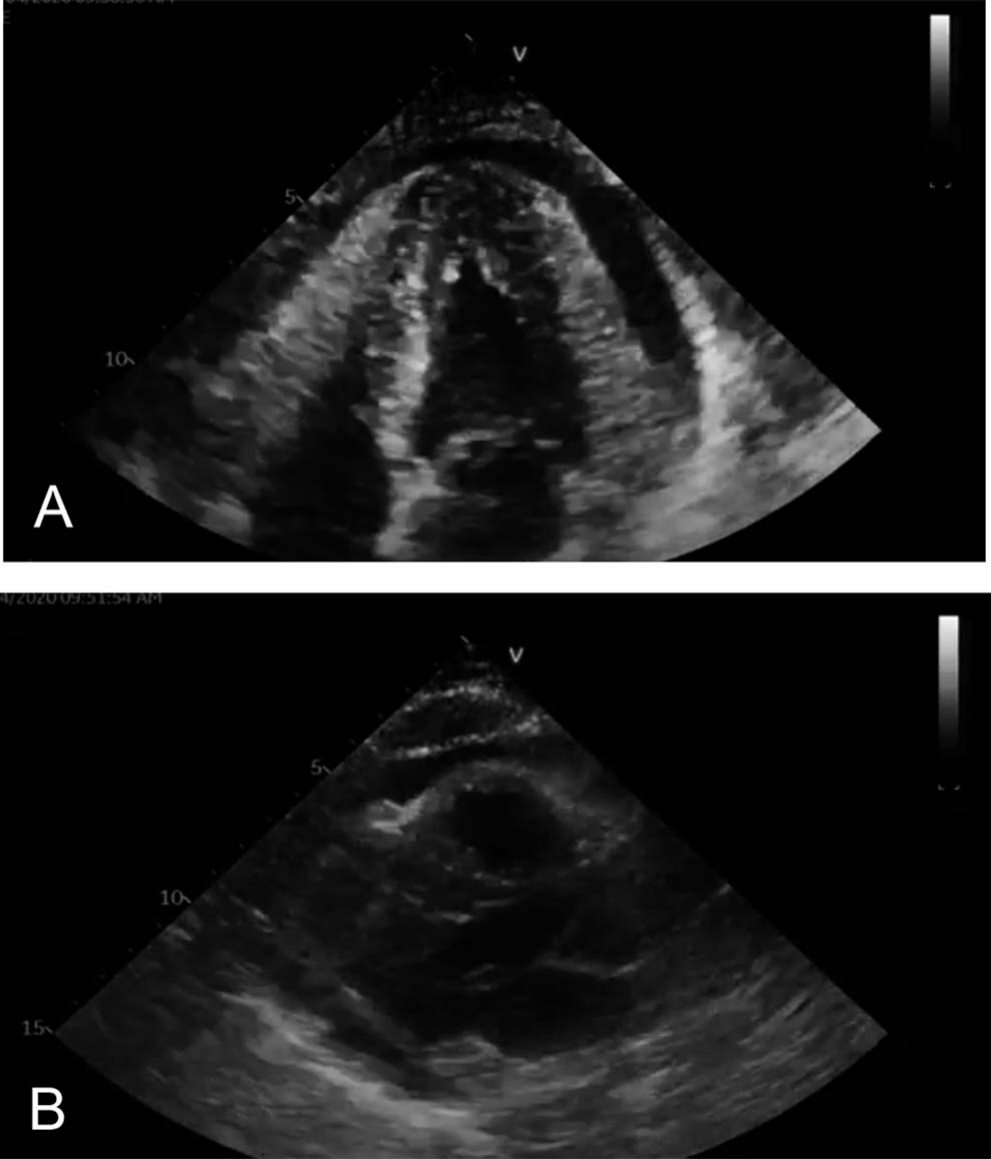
Follow-up echocardiogram in (A) 4-chamber view and (B) parasternal long axis view shows improved ejection fraction of 50%, moderate pericardial effusion, and recovery of segmental wall motion abnormalities.
